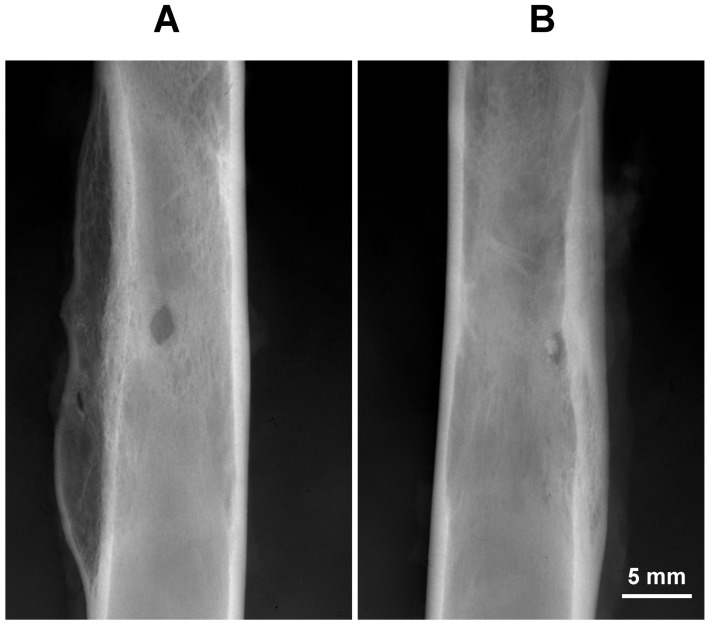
Representative mid-shaft radiographs of two femurs 60 days after the surgery. Note in both radiographs how the shape of hole changes from round (original) to ellipsoidal. Note also how the control hole (A) has a greater size than that of a BGSr filled hole (B).
